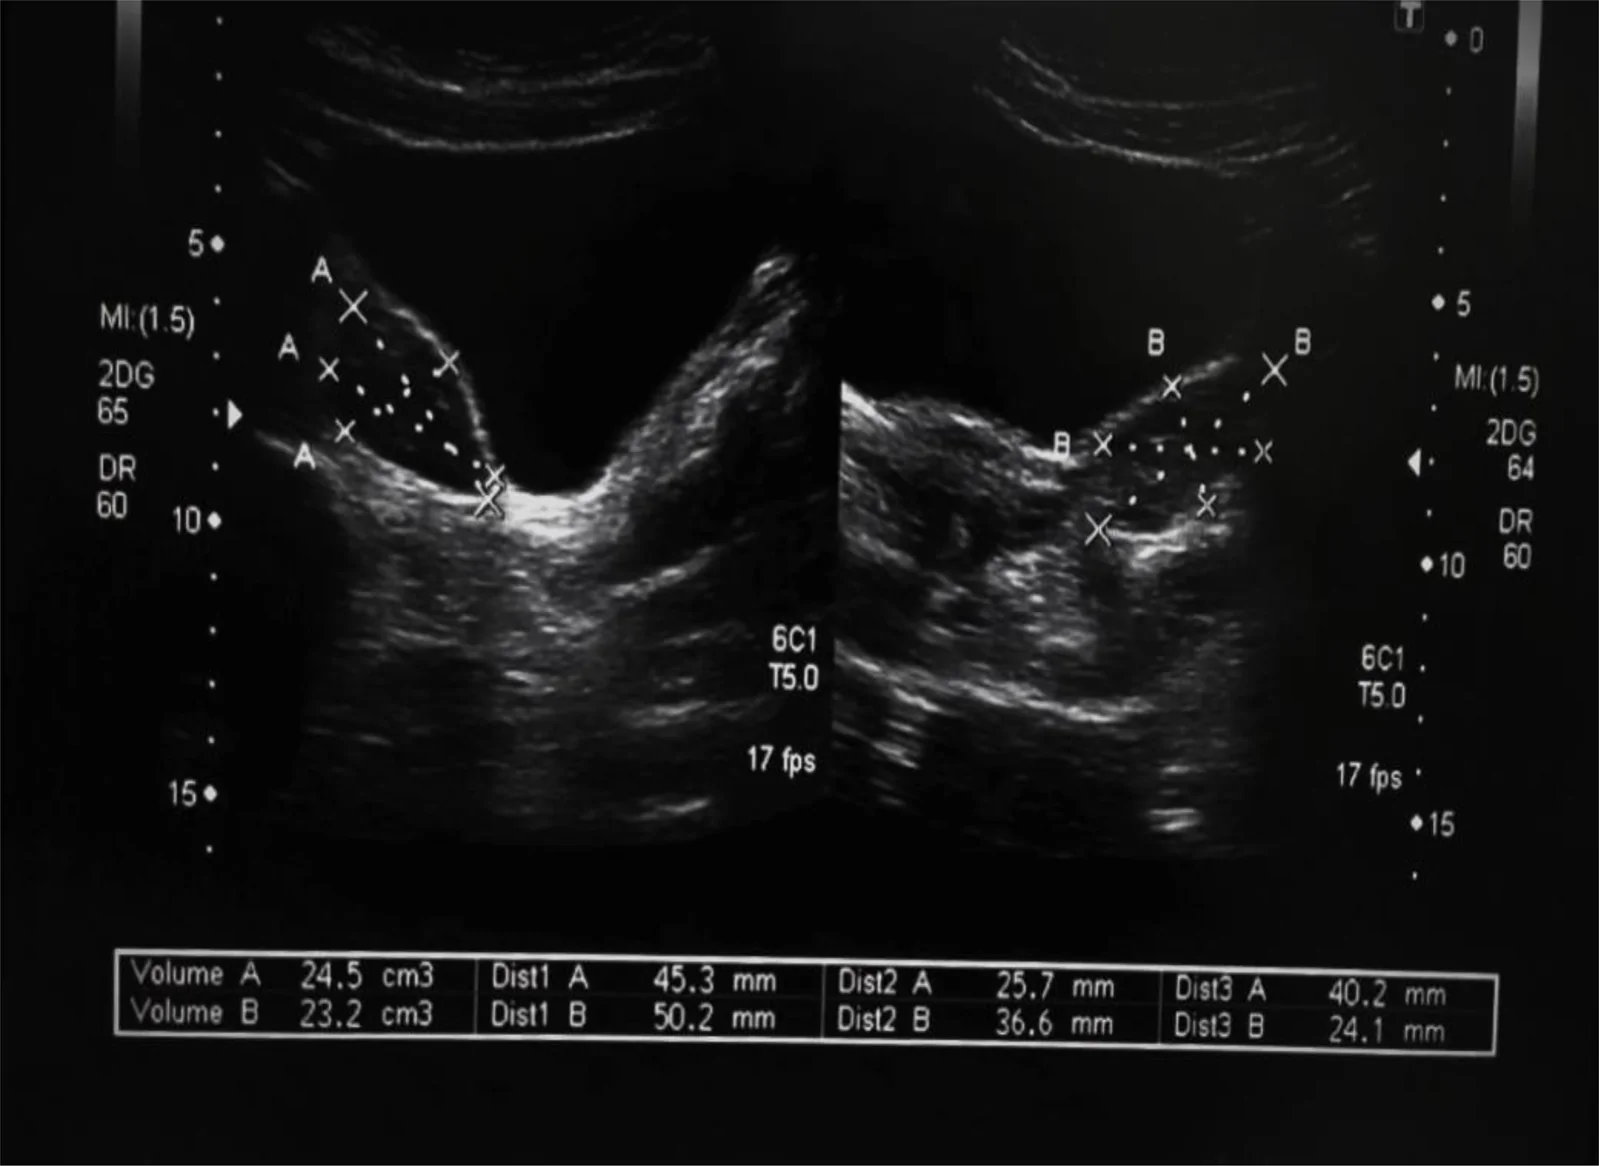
Transabdominal ultrasound of bilateral polycystic ovaries in a 27-year-old woman with high ovarian volume.
